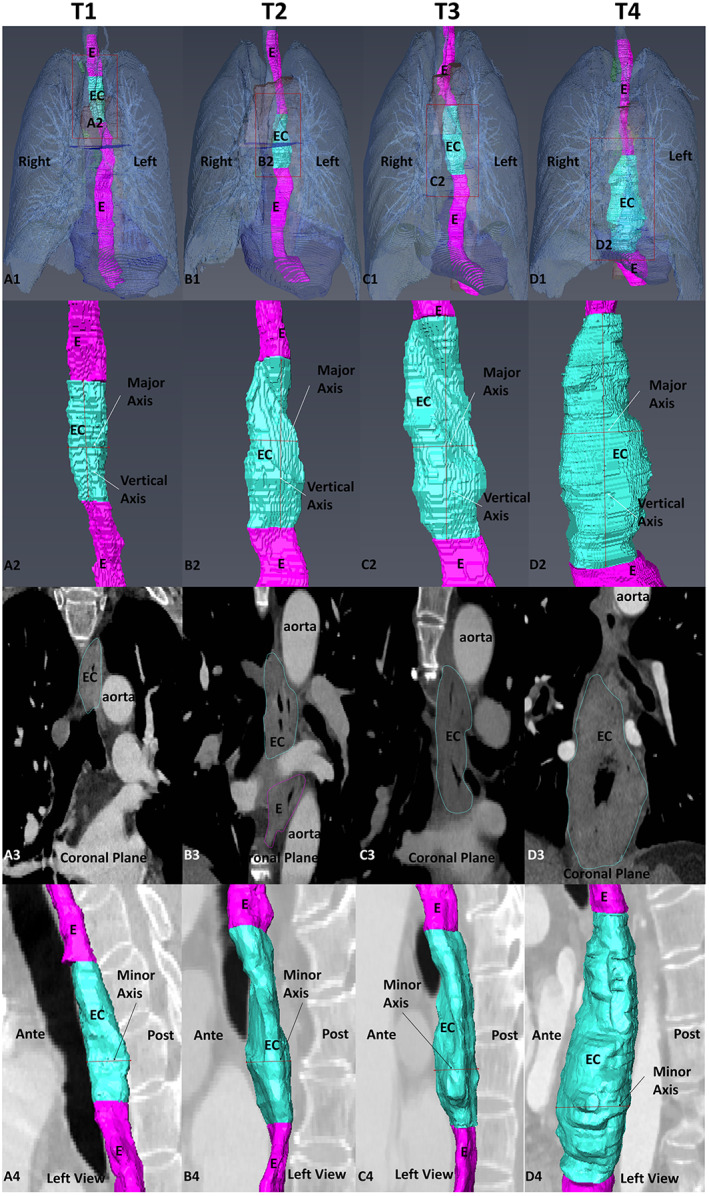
Measurement of tumour roughness and major and minor axis at different T‐stages of EC. A1–D1: 3D reconstructed models; A2–D2: local magnifications, which can determine the roughness and measure the length of the major axis and vertical axis of the EC; A3–D3: coronal CTA images of the tumour area, which can easily show the roughness of the tumour and its position relative to surrounding tissues; A4–D4: sagittal images of the reconstructed tumour superimposed on normal oesophagus (left‐side view), mainly used to measure the minor axis of the tumour. E, normal oesophagus, EC, oesophageal cancer.
